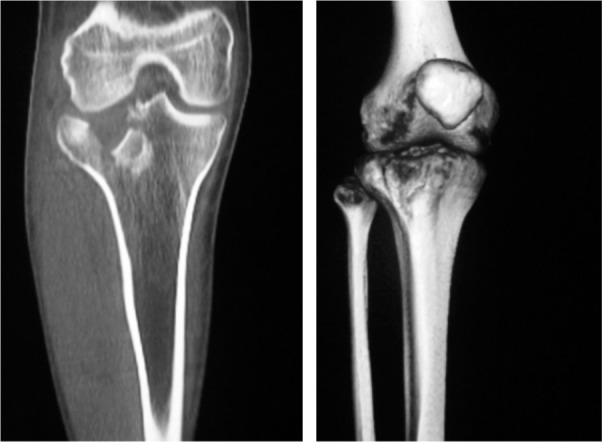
Pre-operative three-dimensional reconstruction CT scan showing Schatzker type II split depression fracture.
